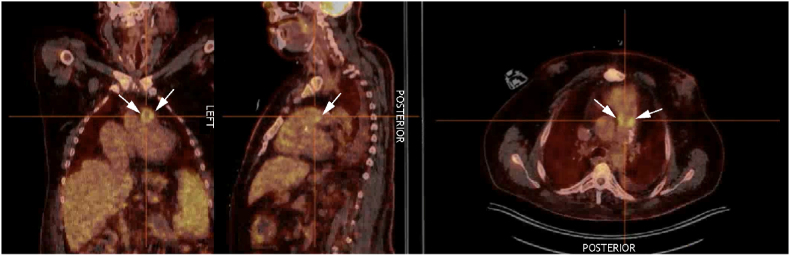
PET-CT scan showing FDG uptake in the pulmonary prosthetic valve (white arrows) suggestive of endocarditis.
